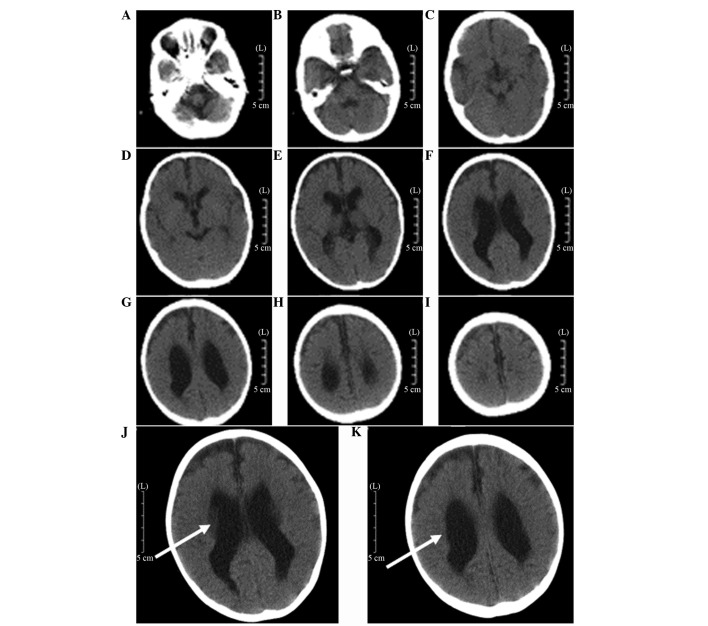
A 2-year old male premature infant with a gestational age of 34 weeks. (A–I) Computed tomography films subsequent to admission. In particular, (E–H) display the different degrees of the periventricular leukomalacia (PVL) manifestations and (F and G) are the more marked among these. (J and K) are amplification of figures F and G, respectively. The body of bilateral ventricles was largely expanded, the caudomedial part and occipital edges were not smooth, and the surrounding white matter was reduced (arrows).
